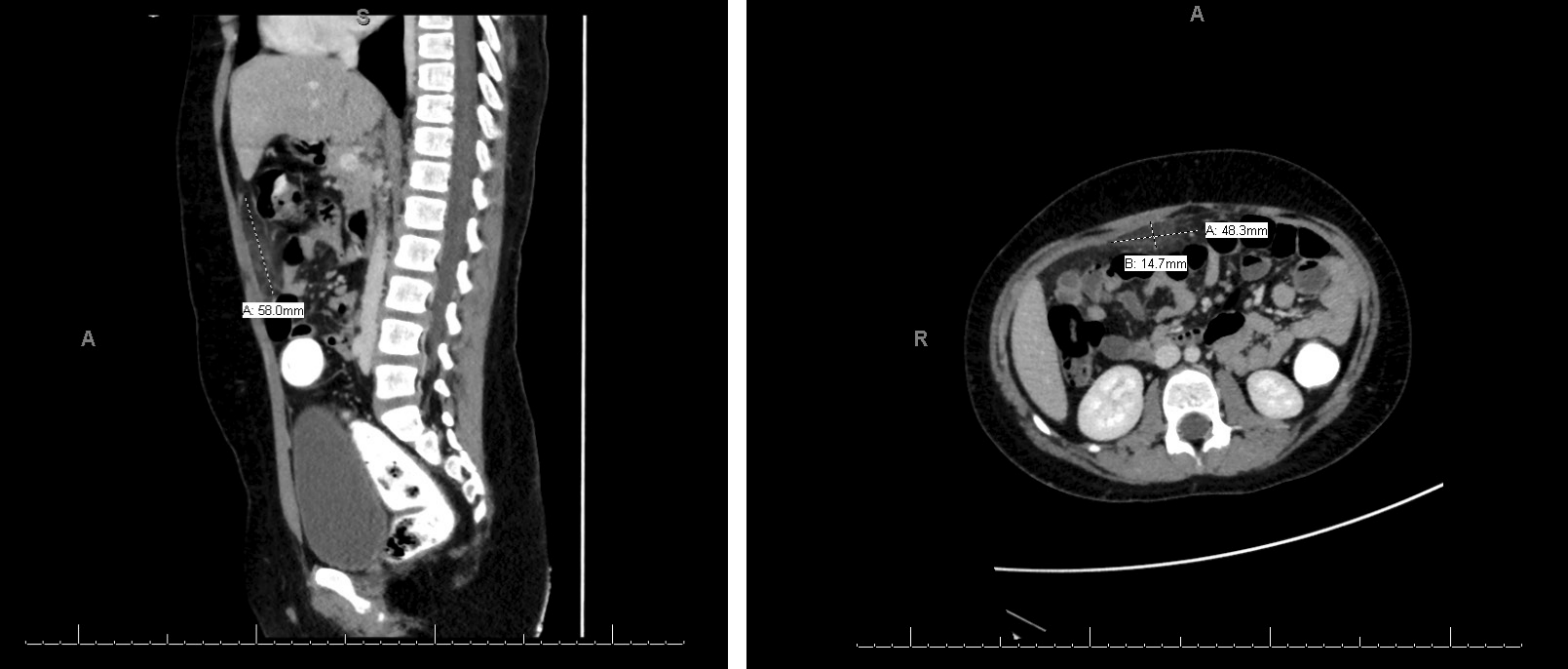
Sagittal and transverse CT scans showing an adherent omental mass on the anterior abdominal wall measuring approximately 5.5 × 1.7 cm.
